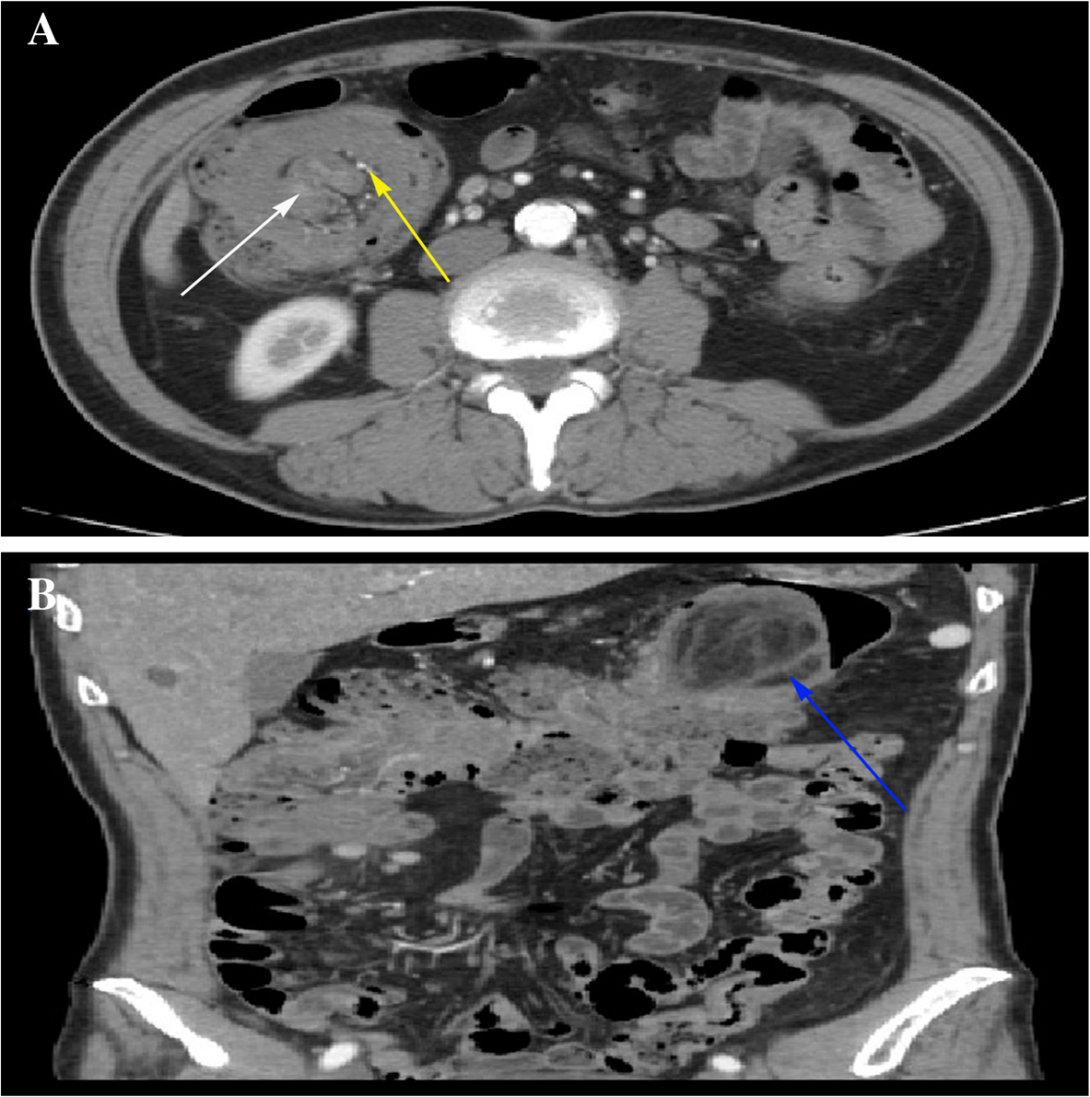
Transverse and coronary contrast-enhanced computed tomography (CECT) of the abdomen. (A) Transverse CECT showed the bowel intussusception (white arrow) entered the intestinal lumen on the right middle abdomen. Enhanced blood vessel (yellow arrow) also entered the intestinal lumen along with the bowel. (B) Coronary CECT showed that the tumor was located at the splenic flexure of the colon (blue arrow) and was accompanied by local intestinal expansion.
